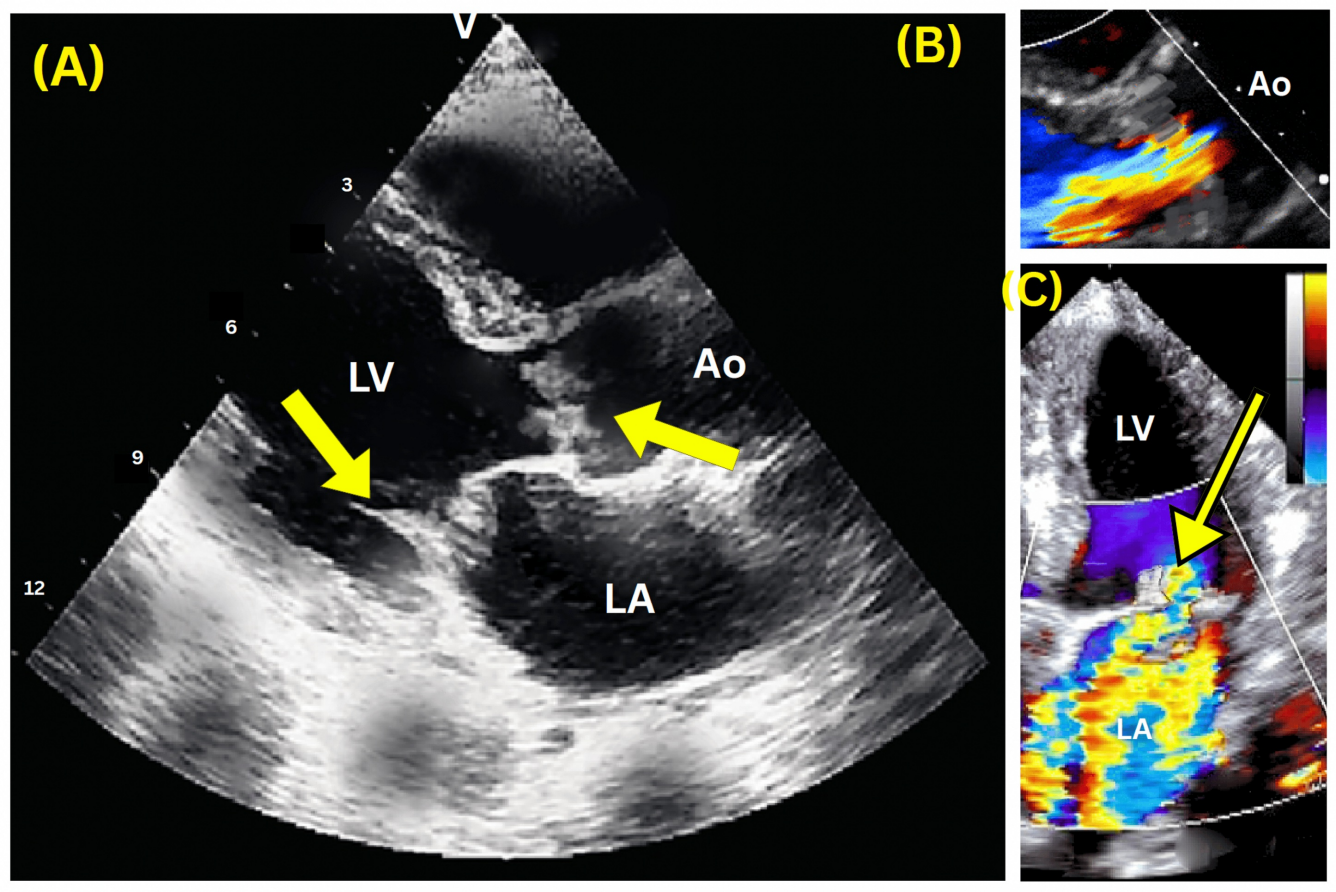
Transoesophageal Echocardiography (A) Two-dimensional transoesophageal echocardiography (TOE) image showing vegetations on the mitral and aortic valves, with the left ventricle (LV), left atrium (LA), and aorta (Ao) labeled. Yellow arrows indicate the presence of vegetations on the mitral valve and the aortic valve.
